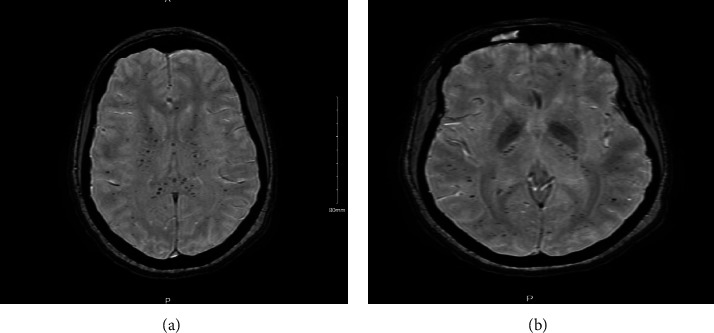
MRI: axial susceptibility weighted sequence showing innumerable small foci of low signal throughout the cerebral hemispheres, brainstem, and cerebellum most consistent with microhemorrhages.
